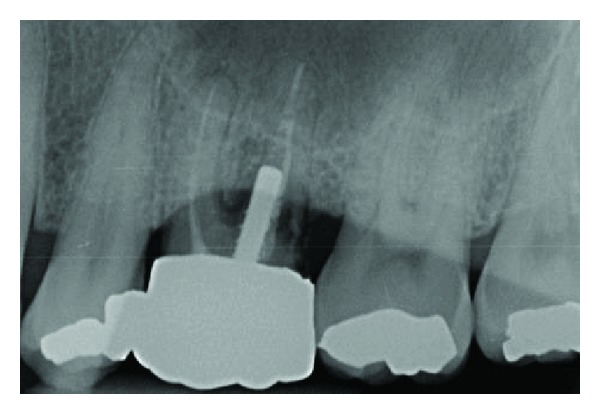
Initial X-ray examination.
